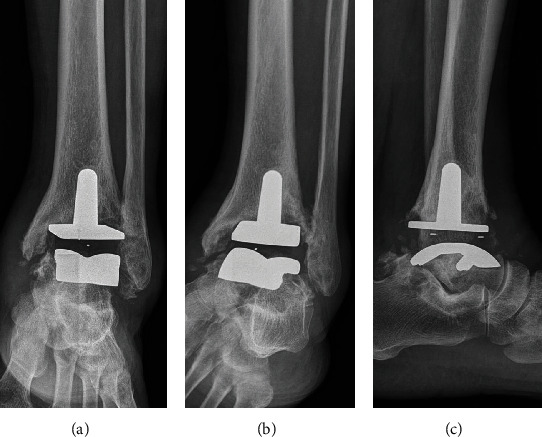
Six months after the TAA. (a, b) The radiological assessment revealed a progressive radiolucent line and osteolysis of the tibial component. (c) A lateral radiograph showed subsidence and a continuous radiolucency around the entire talar component.
